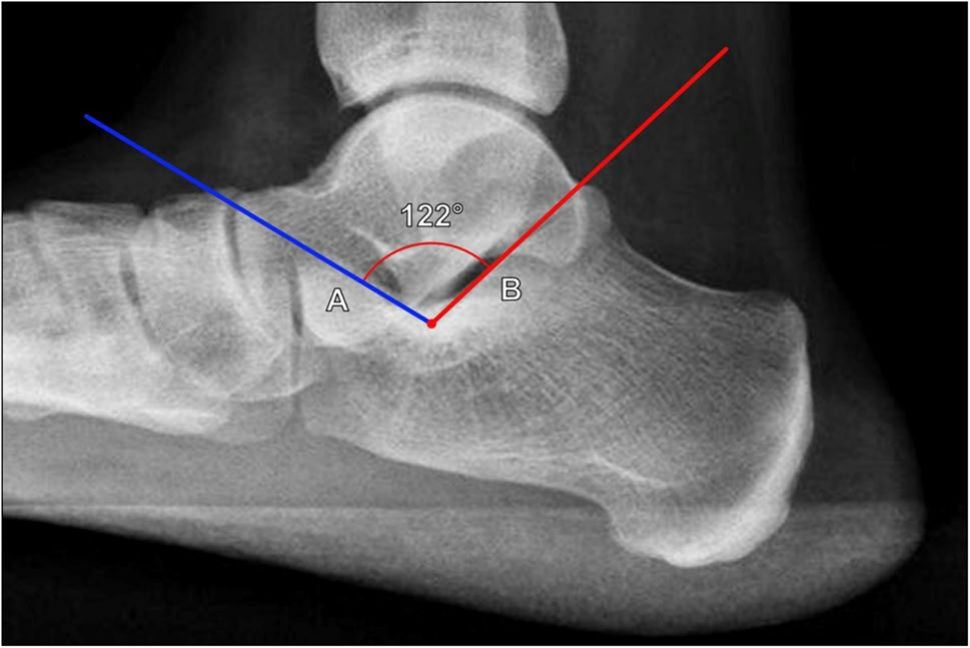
Measurement of Bohler’s angle on weightbearing lateral radiograph of the foot. The angle is formed between a line extending from the tip of the anterior calcaneal process to the superior margin of the posterior calcaneal facet (A, blue line) and a line extending from the posterior calcaneal facet to the superior margin of the calcaneal tuberosity (B, red line). Generally accepted normal values range from 25° to 40°, and values < 25° suggest depression of the calcaneal articular surface with more severe deformities
